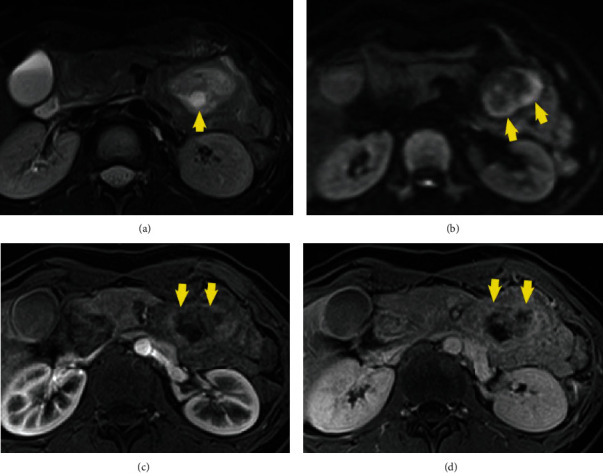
Magnetic resonance imaging (MRI) showing high- and low-signal intensities in the tumor on T2WI (a, b) and no enhancement during the arterial phase (c). The surface of the mass gradually enhanced during the delayed phase (d).
